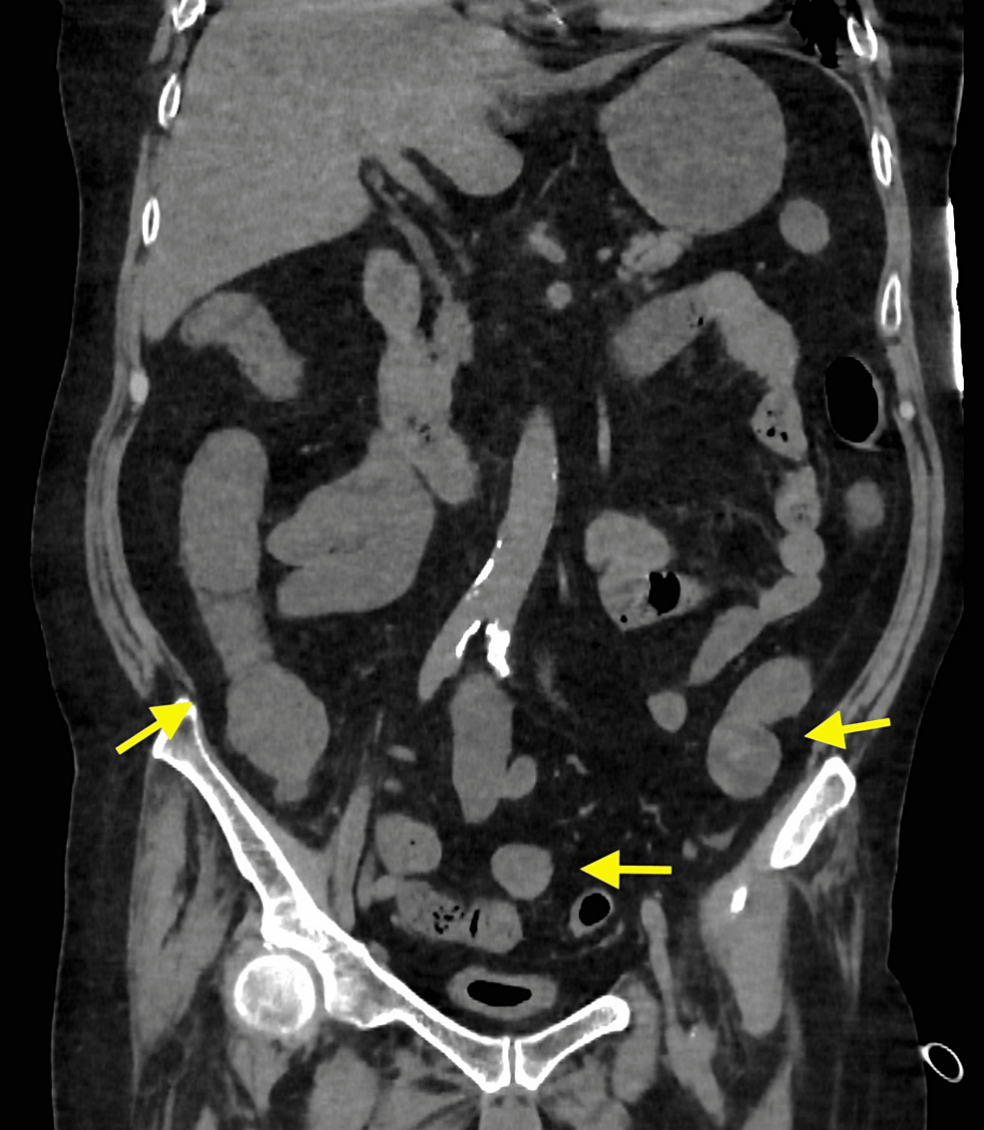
Computed tomography (day 16). A computed tomography scan revealed bowel wall thickening (yellow allows) and fluid retention, suggesting intestinal ischemia. Contrast was preferred, but was not performed due to renal failure.
